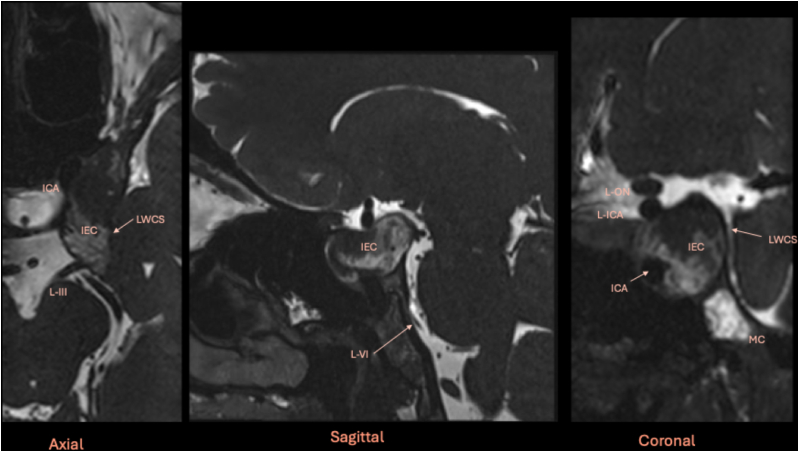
Preoperative multiplanar MRI study with CISS sequences. The pure intracavernous epidermoid cyst (IEC) is clearly located within the cavernous sinus (CS), displacing the internal carotid artery (ICA) anteromedially and laterally bowing the lateral wall of the CS (LWCS), which contains the left third cranial nerve (L-III). *Abbreviations*: ICA: internal carotid artery; MC: Meckel's cave; L-ON: left optic nerve; L-VI: left sixth cranial nerve.
